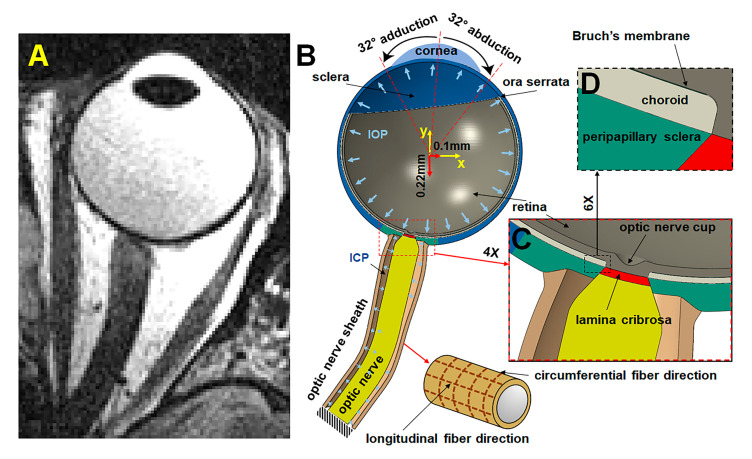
MRI and hemisymmetric eye model. (A) Axial MRI. (B) Hemisymmetric model in ABAQUS. (C) Magnified view of the optic nerve head region. (D) Further magnified view highlighting Bruch’s membrane, situated between the retina and choroid.
